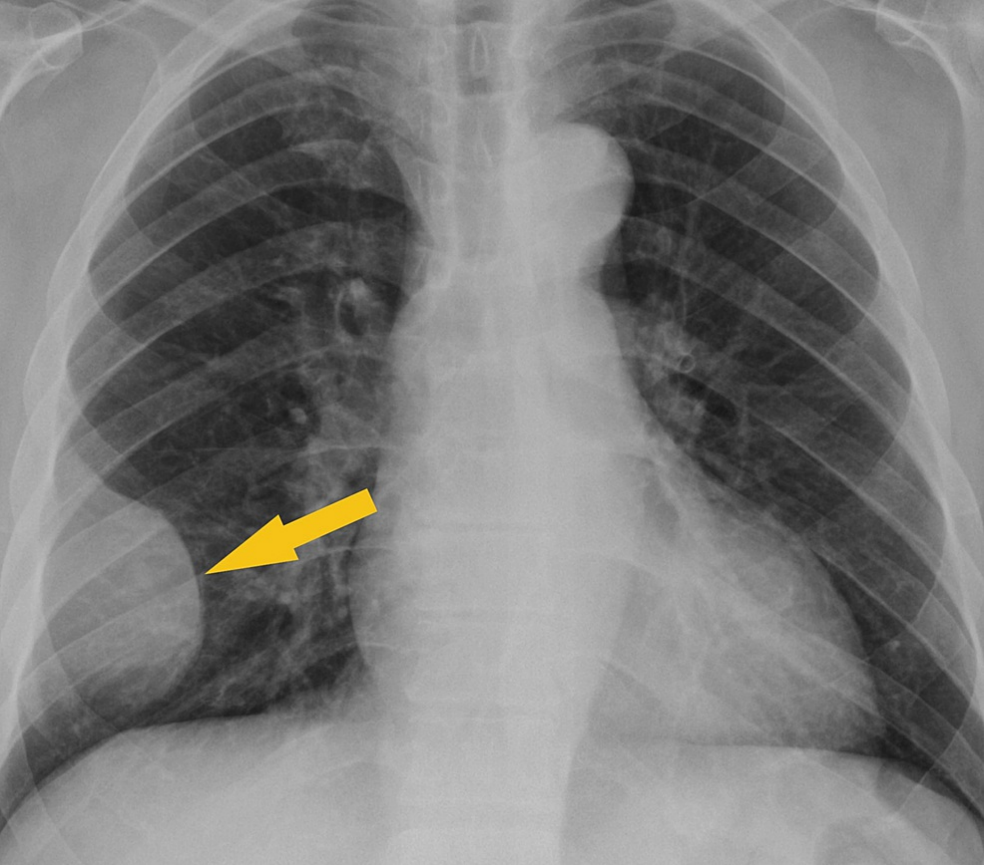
Chest X-ray shows a low-density soft tissue structure located at the periphery of the right hemithorax, measuring approximately 9 cm in size
